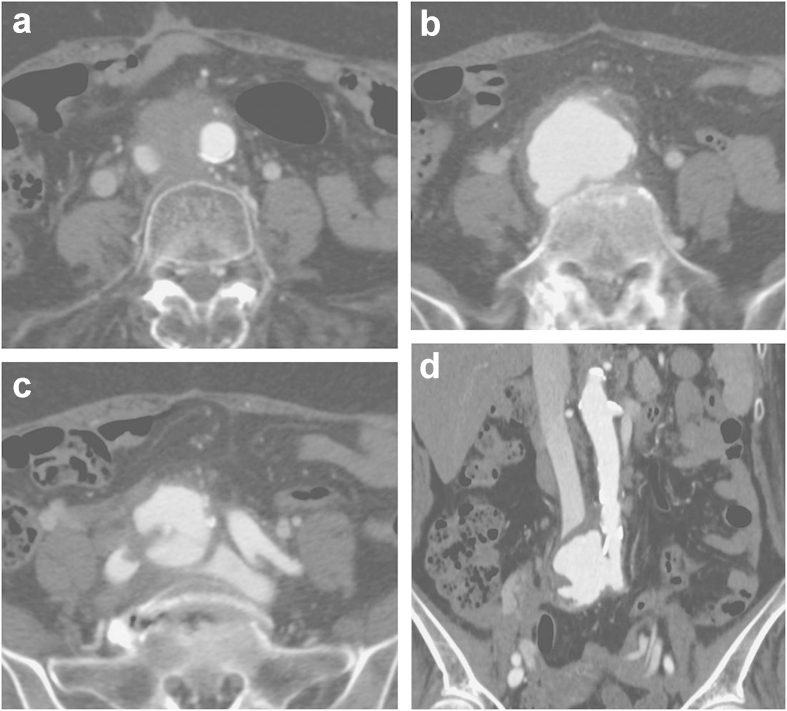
Contrast-enhanced CT imaging. a) Contrast-enhanced CT at the level of the lower abdominal aorta. A nonenhancing mass is recognized in the aortocaval space. The IVC is compressed and narrowed. b) Contrast-enhanced CT at the level of the terminal aorta. A large aneurysm with an irregular shape is recognized. c) Contrast-enhanced CT at the level of the CIA. The aneurysm and normal left CIA are visualized. Early venous filling of the left CIV and filling defect of the right CIV are recognized. d) Coronal multiplanar reconstruction contrast-enhanced CT image. An aneurysm arising at the terminal aorta and compressed IVC are recognized.
